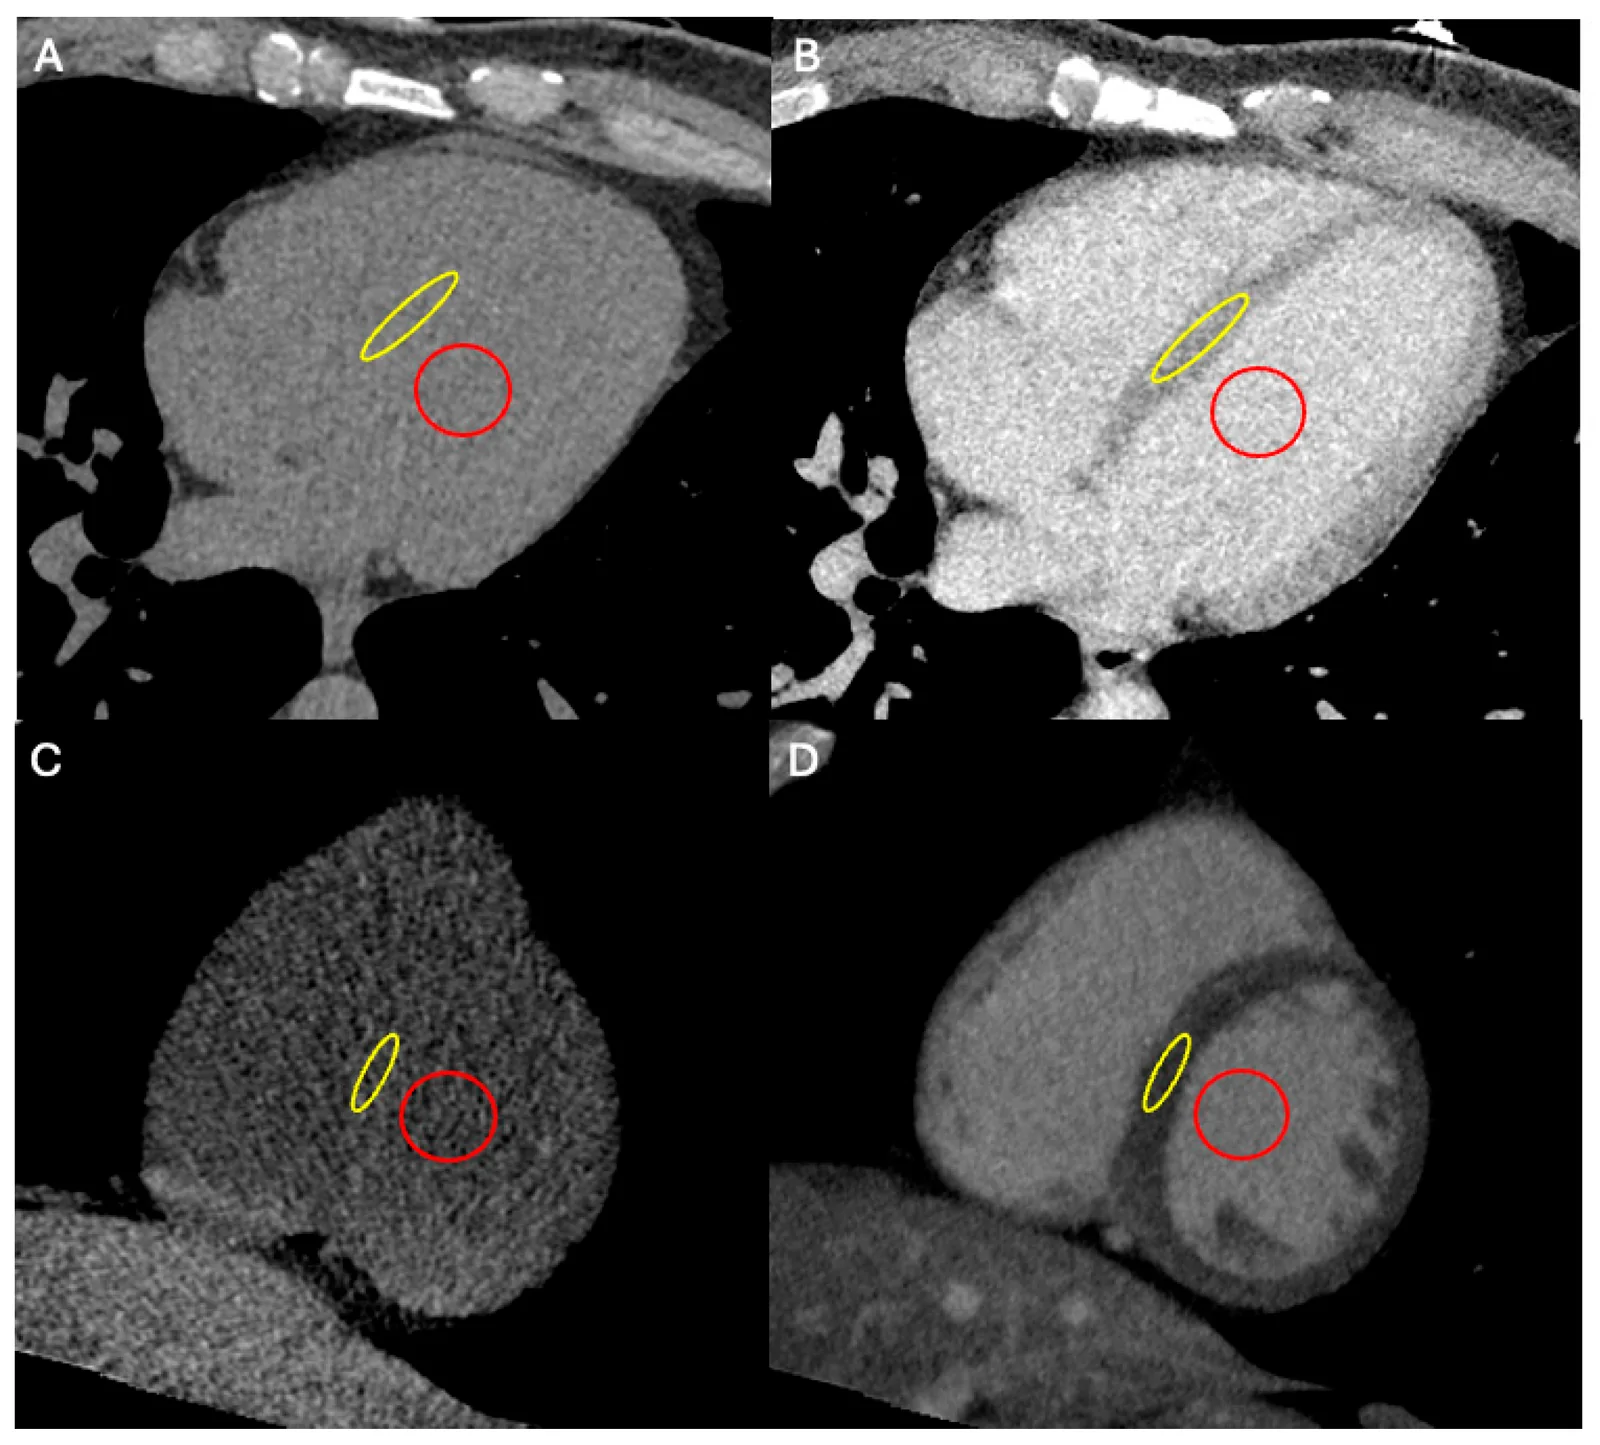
ECV measurement using the subtraction method in single-energy computed tomography (SECT). Regions of interest (ROIs) were initially drawn in the mid-ventricular septum (yellow ROI) and in the left ventricular blood pool (red ROI) on the late iodine enhancement (LIE) post-contrast scans in four-chamber (B) and mid-ventricular short-axis (D) views. To ensure constant ROI dimensions and precise anatomical location, these ROIs were subsequently copied onto the exact same locations on the co-registered non-enhanced baseline scans (A,C, respectively). The use of automatic, three-dimensional non-rigid image registration is recommended to electronically align the myocardial borders, thereby mitigating spatial misregistration artifacts.
